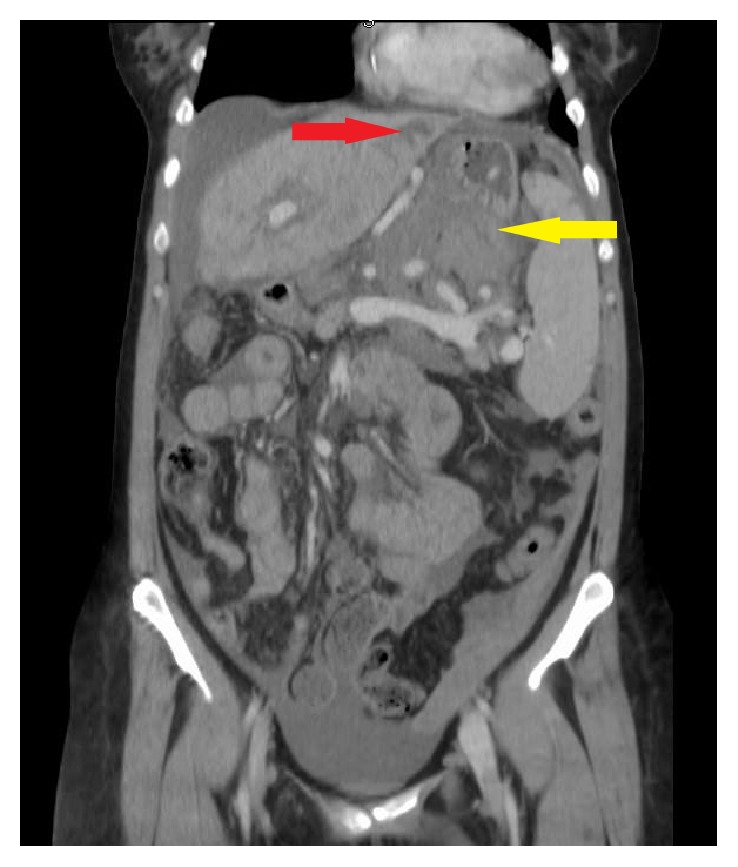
Coronal image from abdominal CT with contrast captures the development of hemoperitoneum (yellow arrow) from splenic varices and hypodensity in left lobe of liver suggestive of cholangiocarcinoma (red arrow).
